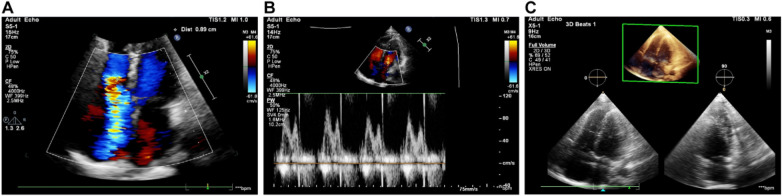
Postoperative follow-up echocardiographic results (August 31, 2025). (A) Color Doppler imaging of the apical four-chamber view; (B) The spectrum Doppler image of tricuspid regurgitation; (C) Two-dimensional ultrasound + three-dimensional ultrasound section.
